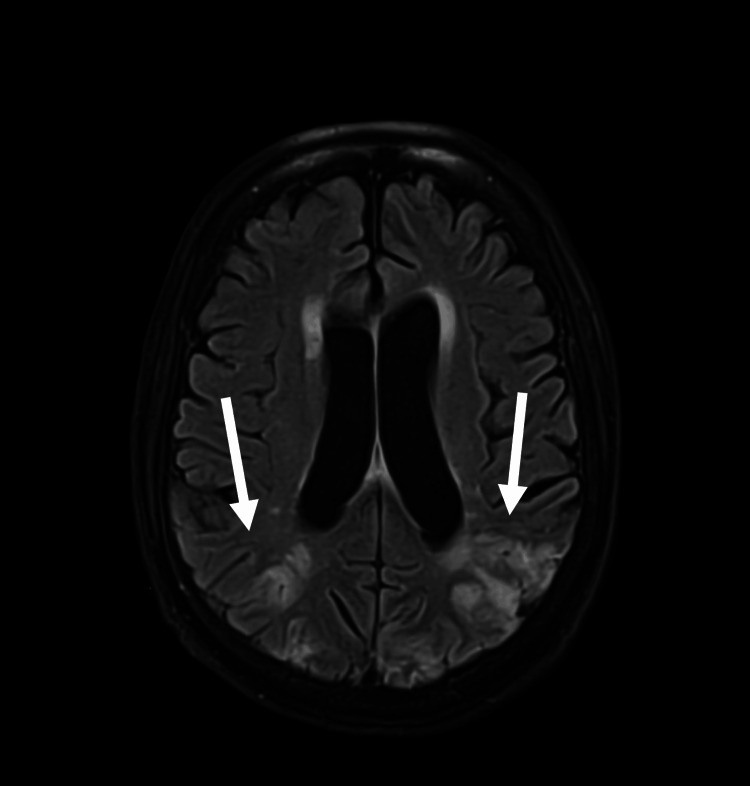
MRI with contrast showing multiple zones of acute ischaemia in the cerebral and cerebellar hemispheres bilaterally. Axial MRI brain with contrast showing multifocal acute ischaemic changes involving both cerebral and cerebellar hemispheres, most pronounced in the posterior watershed territories. (Image obtained from the hospital PACS system with patient consent.) Arrows indicate areas of hyperdensity, confirming multiple acute ischaemic infarcts.
